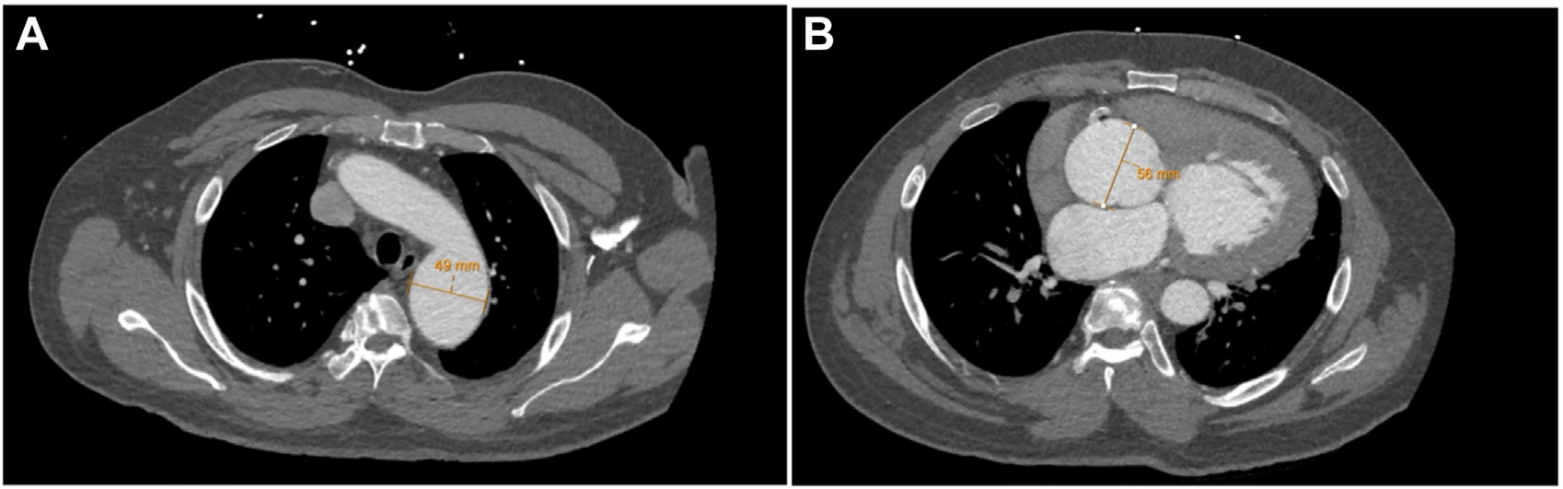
Preoperative contrast-enhanced computed tomography angiography showing measurements of (A) distal arch aneurysm and (B) aortic root aneurysm in axial view.
